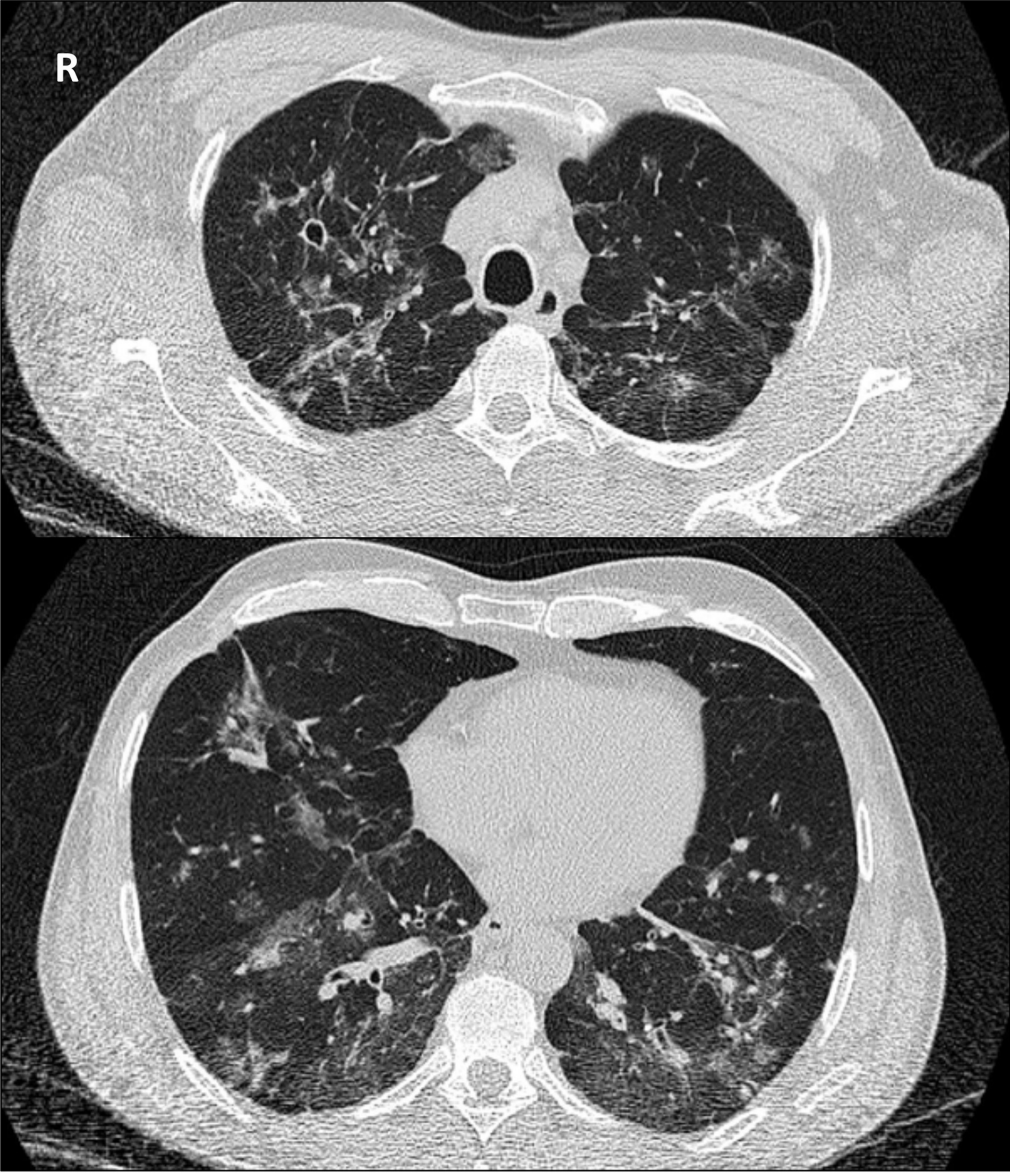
CT scan of pulmonary paracoccidioidomycosis with bilateral small nodules, ground glass changes, and a small cavity in the right upper lobe. CT, computed tomography.
